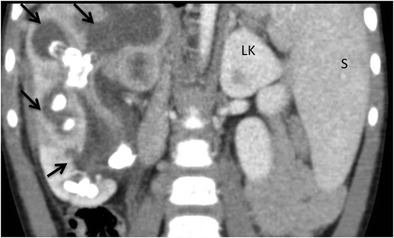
Diffuse XPN. Contrast-enhanced nephrogenic phase coronal reconstruction CT shows right kidney with multiple low attenuation round masses (*black arrows*) corresponding to either dilated calyces or focal areas of parenchymal destruction with numerous calculus in calyces. *LK* left kidney, *S* spleen
